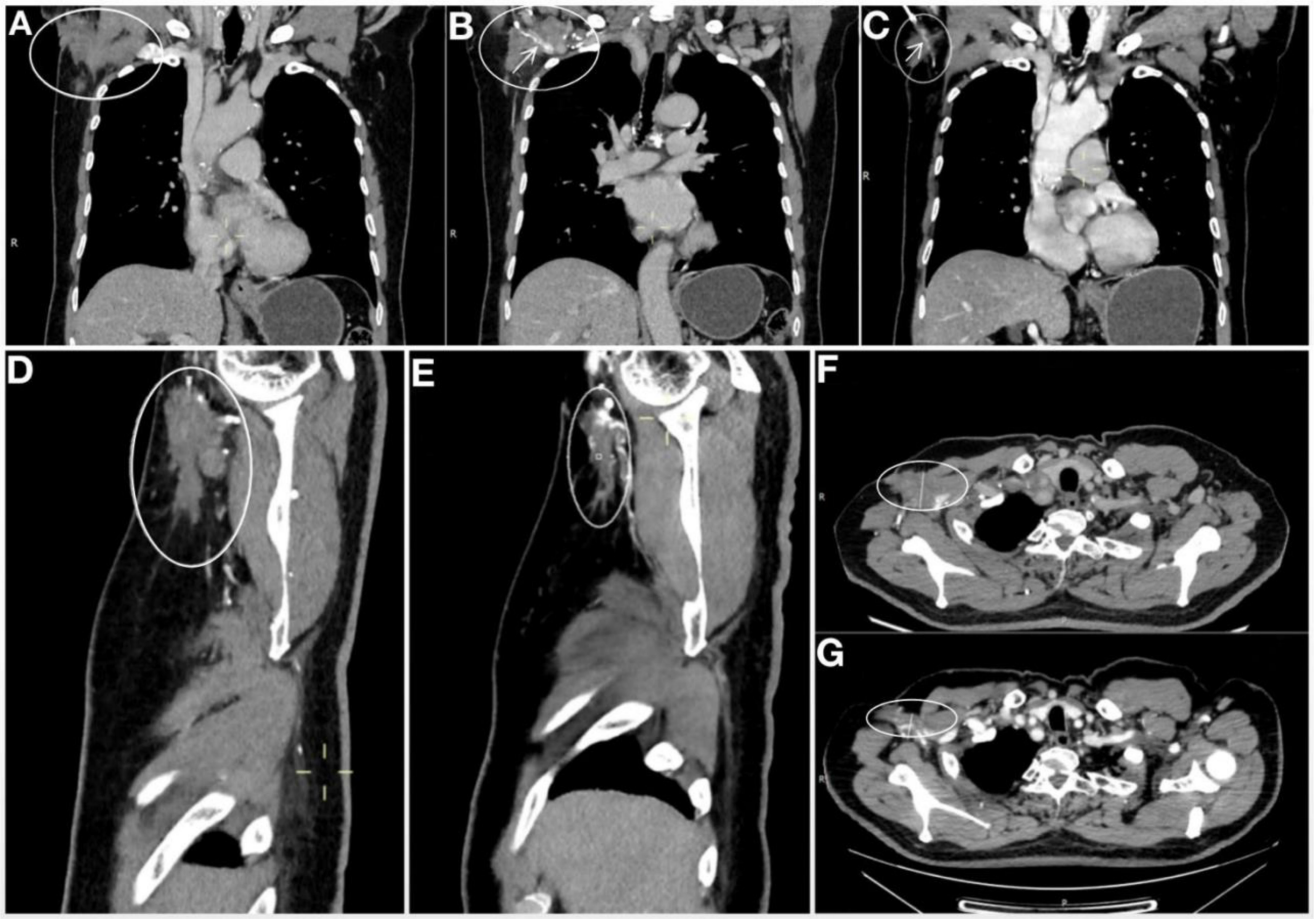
Comparison of right axillary CT after 2 cycle system treatment and before operation. (A-C) are the coronal plane imagine. (A) Imagine after 2 cycles of systematic treatment. (B) Imagine after 2 cycles of systematic treatment, demonstrating the mass tightly encircled the axillary vein. (C) Pre-operative image (Image after 8 cycles +6 cycles of systemic treatment), Compared with Figures 3A, B, the tumor was significantly reduced, and the tumor could be seen to surround part of the axillary vein. (D) Sagittal plane Imagine after 2 cycles of systematic treatment. (E) Sagittal plane Imagine pre-operative (after 8 cycles +6 cycles of systemic treatment). (F) Horizontal plane Imagine after 2 cycles of systematic treatment. (G) Horizontal plane Imagine pre-operative (after 8 cycles +6 cycles of systemic treatment). Imagine of sagittal plane and horizontal plane manifest that the tumor was significantly reduced before operation compared with post 2 cycles of systemic treatment, as well as coronal plane. The arrow marks the axillary vein, the oval marks the mass.
